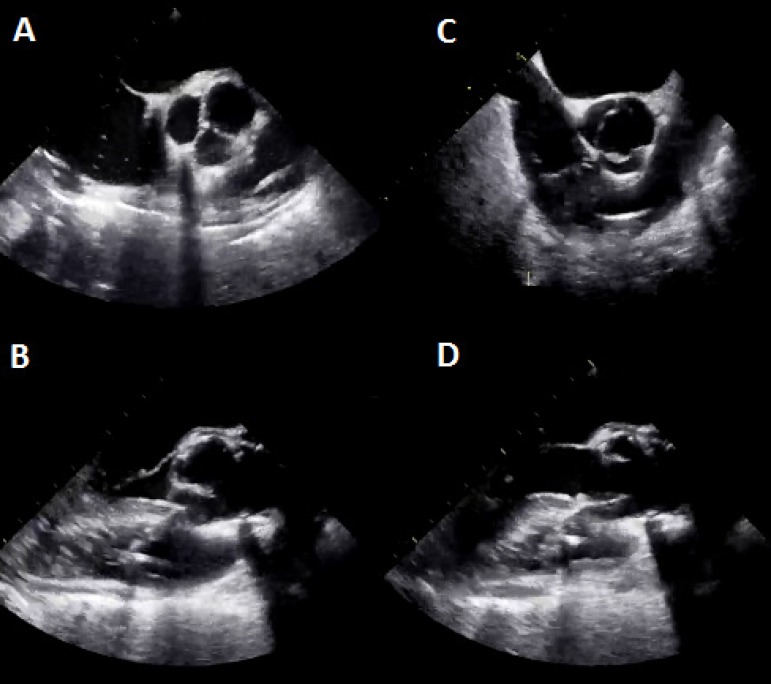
Echocardiographic pictures after the surgical procedure. A and B
